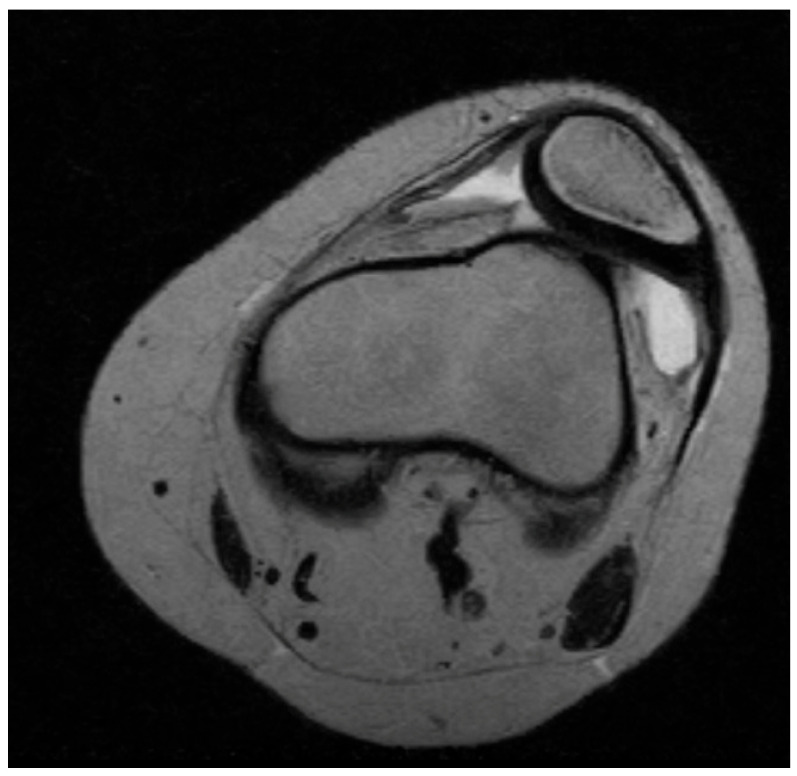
Axial MRI in extension of an 8-year-old girl with habitual patellar dislocation. A patellar subluxation and patellar tilt, as well as trochlear dysplasia, are easily observed.
